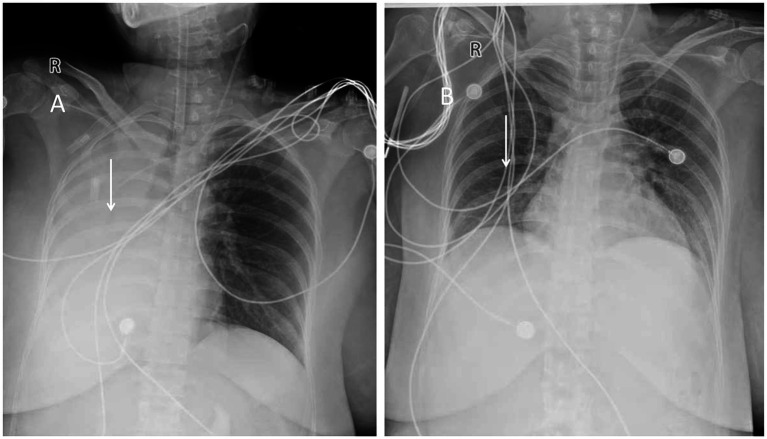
(A) Chest X-ray showing right lung atelectasis. (B) Follow-up chest X-ray showing near-normal appearance.
